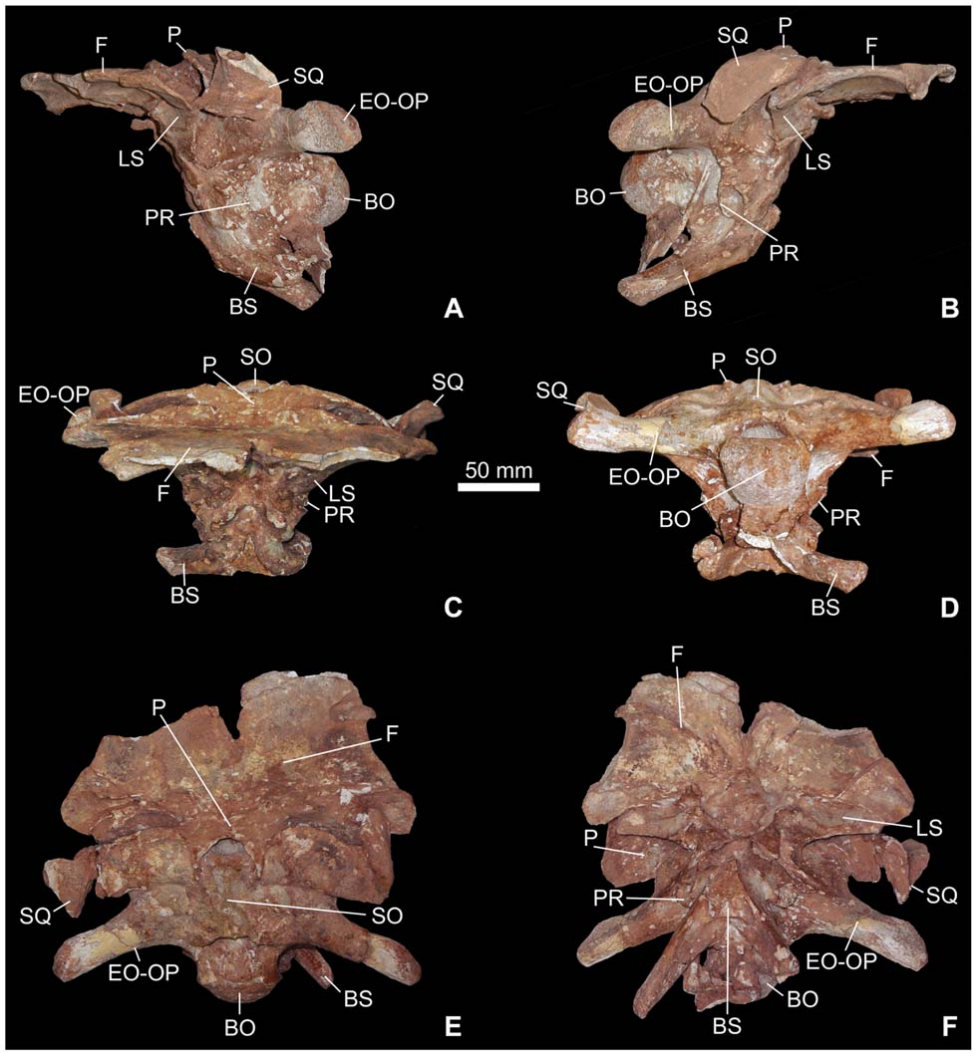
Photographs of the braincase of the sauropod dinosaur *Spinophorosaurus nigerensis* (GCP-CV-4229) from the Jurassic of Aderbissinat, Niger; in left lateral (A), right lateral (B), rostral (C), caudal (D), dorsal (A), and ventral (B) views. Abbreviations herein, in Figure 2, and Figure 3A–D: BO, basioccipital; BP, basipterygoid process; BS, basisphenoid; BT, basal tuber; C, columella; CA, crista antotica; CAR, carotid artery; CO, crista otosphenoidalis; CP, capitate process; CPC: craniopharyngeal canal vestigial pit; CT, crista tuberalis; CVCM, dorsal-head/caudal-middle-cerebral vein system groove; EO-OP, exoccipital-opisthotic; F, frontal; FM, fenestra metotica emplacement; FO, fenestra ovalis emplacement; FOM: foramen magnum; III, oculomotor foramen; LS, laterosphenoid; NC: nasal cavity recess; OBF: olfactory bulb fossa; OC, occipital condyle; OCV, orbitocerebral vein foramen; OR: orbital recess; P, parietal; PAF: proatlas facet; PFO, pituitary fossa emplacement; PIN, pineal foramen; PP, paroccipital process; PPF, postparietal fenestra; PR, prootic; PTF: posttemporal fenestra; RVCM, rostral middle cerebral vein foramen; SO, supraoccipital; SQ, squamosal; UTF, upper temporal fenestra; V, trigeminal foramen; VII, facial foramen emplacement; XII, hypoglossal foramen emplacement.
